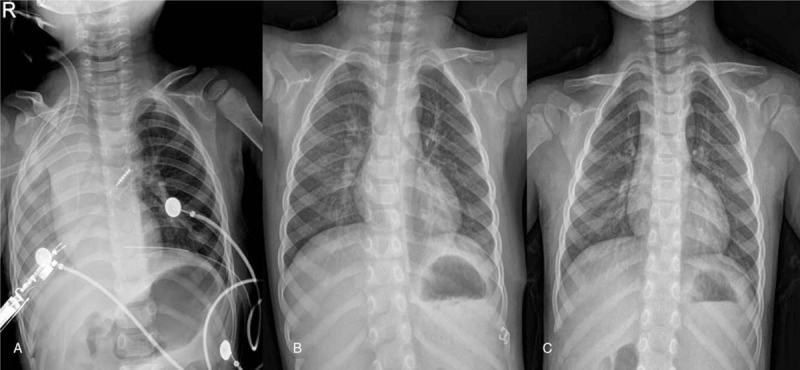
Chest x-rays from immediately after surgery (A), time of discharge (B) and 1 yr follow up (C). The diaphragm is now normal in shape, and normal location of the stomach gas.
